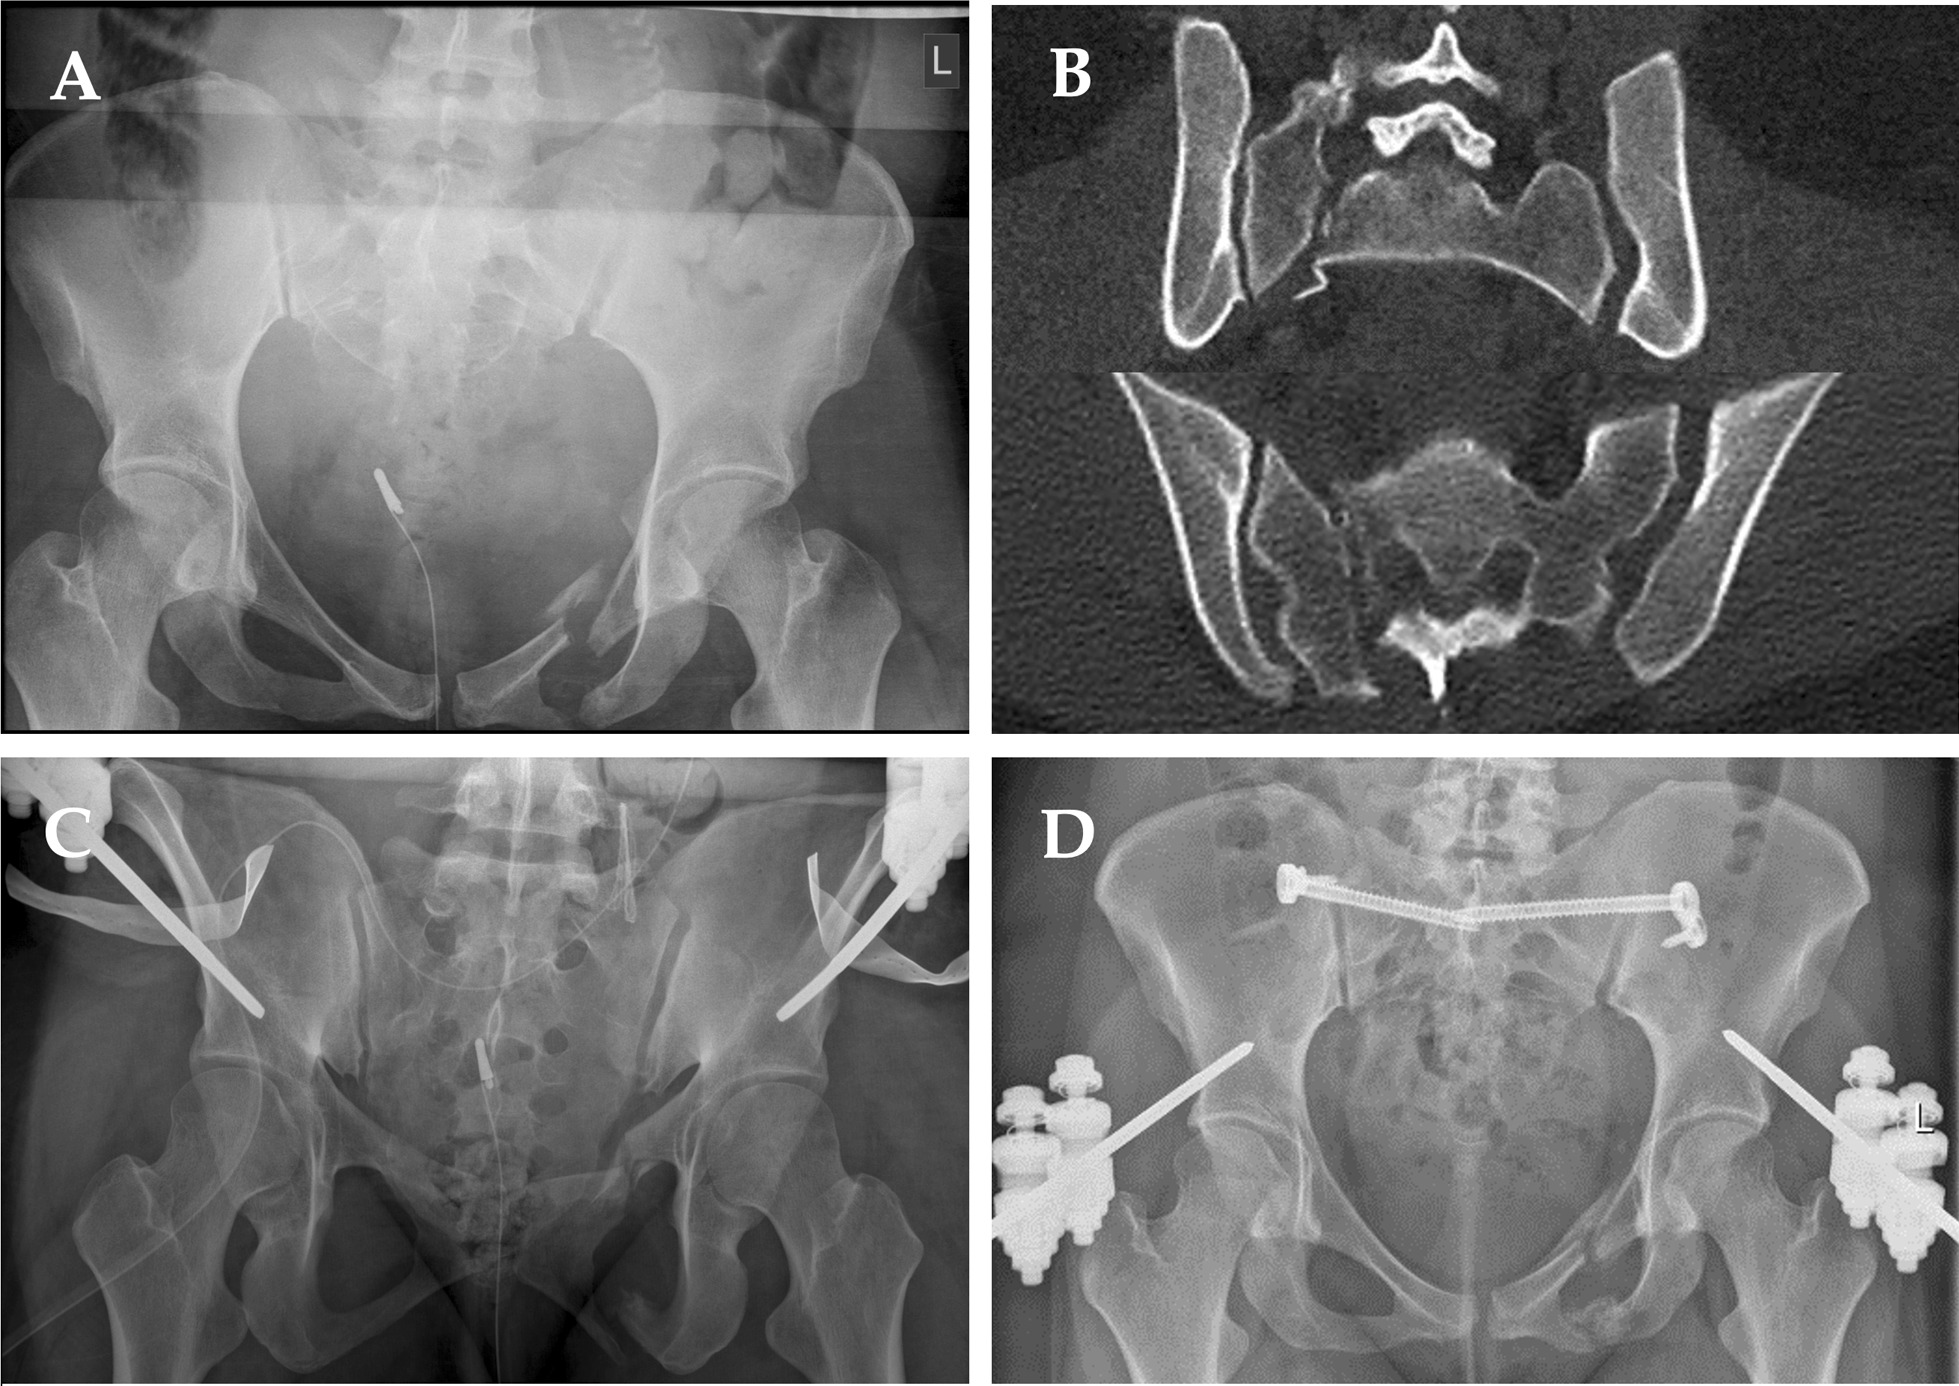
Case of a AO Typ C pelvic fracture (AO 61 C3.2) in a 30-year-old female after car accident. a Initial x-ray of the pelvis in the emergency department showing the injury of the anterior and posterior pelvic ring. b Two slices from the CT-scan showing the fracture of the sacrum on the one and the rupture of the iliosacral joint on the other side. c X-ray control after initial stabilization with a supraacetabular fixateur. d X-ray of the pelvis after stabilization of the dorsal pelciv ring using bilateral iliosacral screws with washer plates
